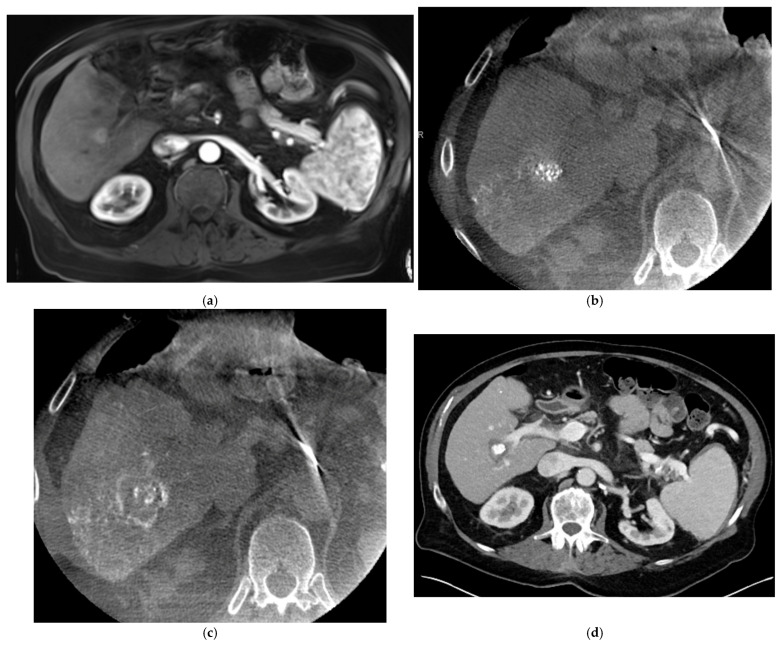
(a–d) 70 year old male with hepatitis C cirrhosis with 1.6 cm hepatocellular carcinoma in segment 5. The patient was not a surgical or transplant candidate due to comorbidities. (a) Pretreatment axial arterial phase MRI showing an enhancing HCC in segment 5. The lesion was invisible on US. (b) CBCT acquired in the same session following Lipiodol injection. (c) Contrast-enhanced CBCT immediately post-ablation in the same session showing an ablative margin. (d) Contrast-enhanced CT 4 months post-ablation with nonviable lesion.
